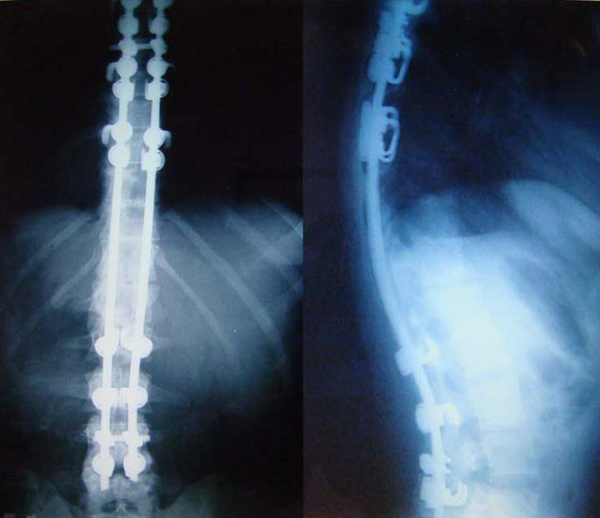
Final radiographs. Posteroanterior and lateral views after the three stages of surgery.
